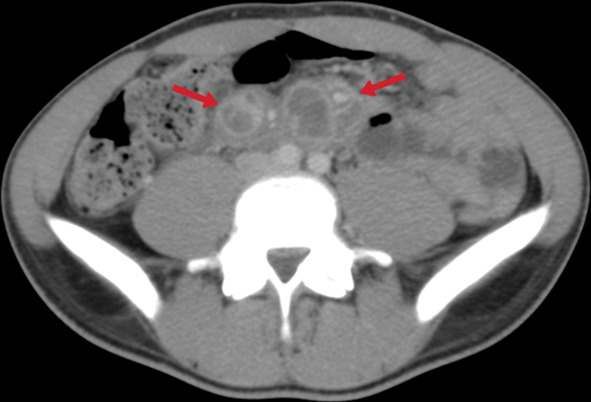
A 19-year-old male. Post-contrast CT image shows multiple mesenteric lymphadenopathies with peripheral enhancement
